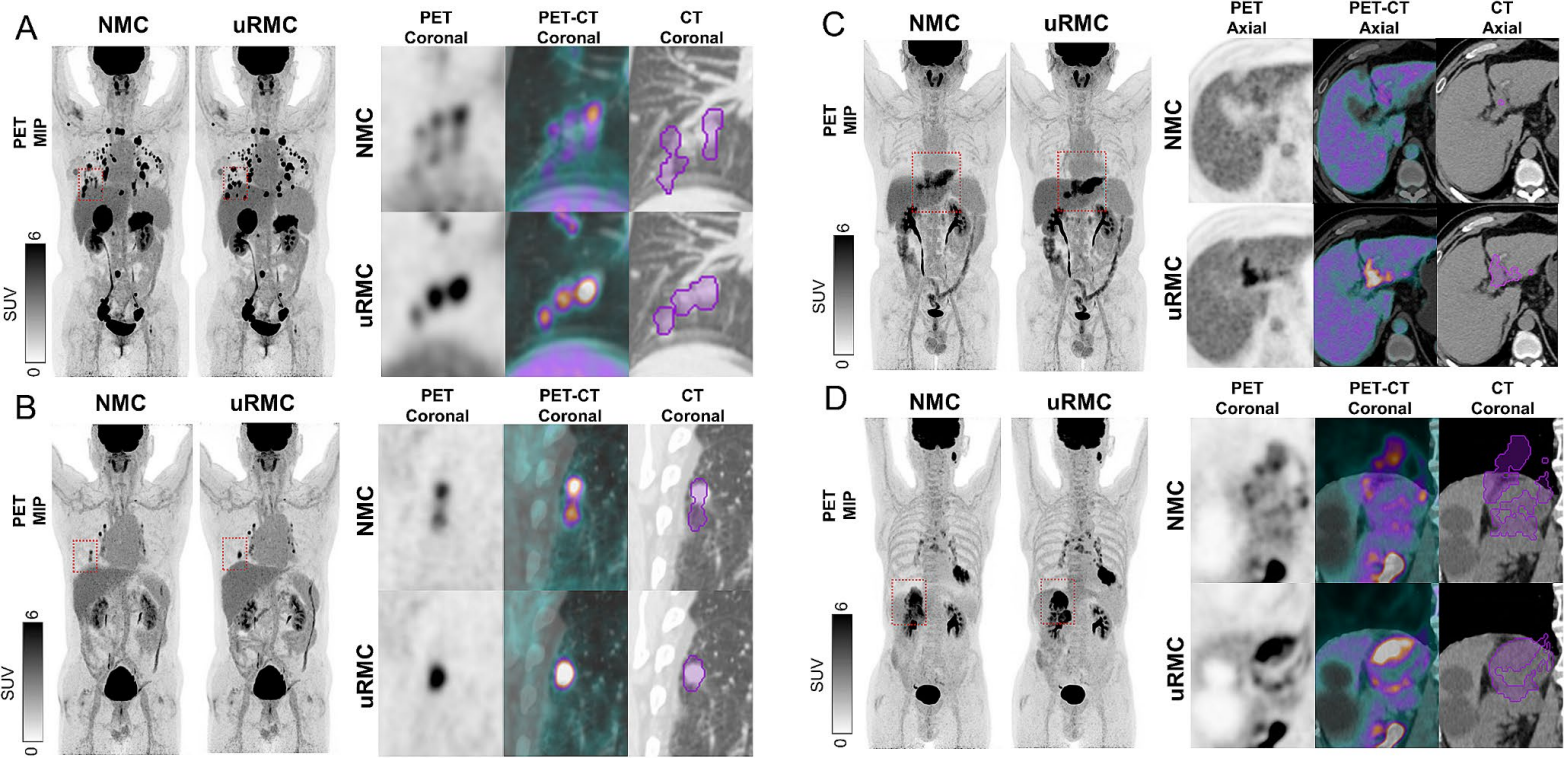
Comparison of NMC with uRMC images. Compared with NMC images, uRMC images show sharper lesion contours, smaller metabolic volume, increase in SUV values, and improved PET-CT alignment. (A) Delineation of multiple lower lung nodules in a patient with diffuse metastatic small cell neuroendocrine carcinoma of the cervix. (B) Double contouring of a lower lung nodule in the NMC images, whereas uRMC images reveal a single nodular contouring. (C) In a patient with hepatocellular carcinoma, an [^18^F]FDG-avid tumor thrombus is clearly seen in the PET and well registered to the portal vein on CT after MC. (D) In a patient with lung cancer, an [^18^F]FDG-avid adrenal metastatic mass is well registered in PET/CT after applying uRMC while NMC images exhibit smeared adrenal uptakes due to respiratory motion. NMC: no motion correction; uRMC: unified respiratory motion correction
